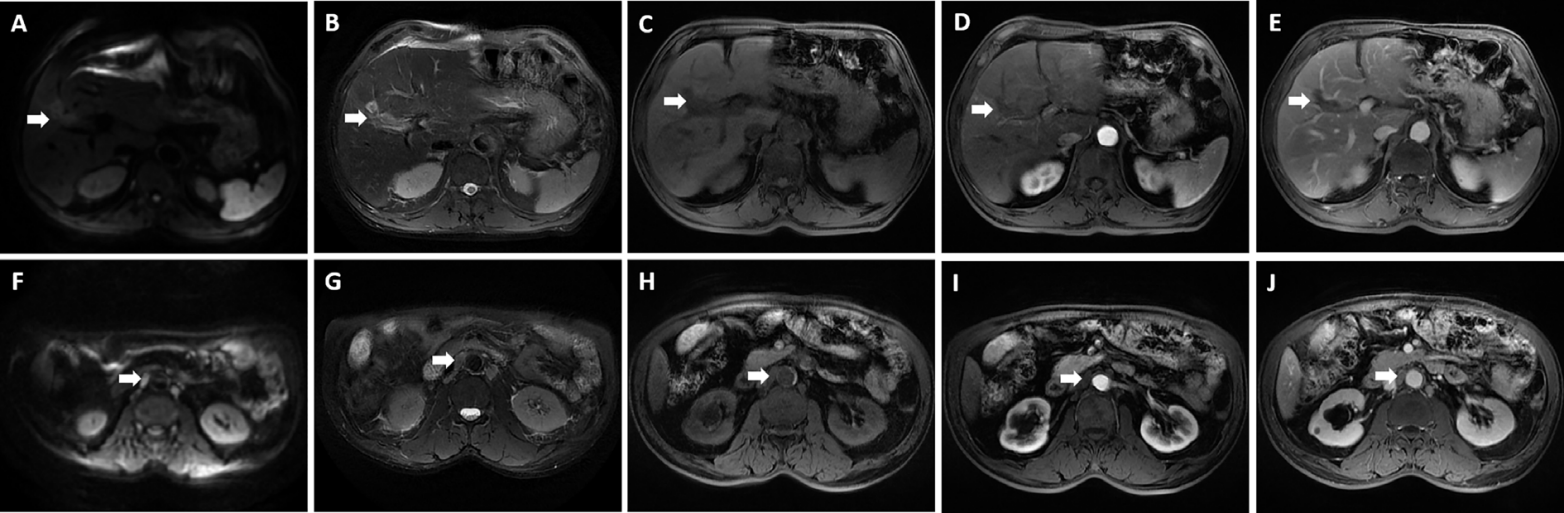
Preoperative magnetic resonance imaging was performed for the patient. The reduced lesion with clear boundary has low signal in T1-weighted imaging, high signal in T2-weighted imaging, and limited diffusion in diffusion-weighted imaging (DWI); enlarged hepatic portal and retroperitoneal lymph nodes were also revealed—the larger one was about 10 mm in diameter. (A, F) DWI; (B, G) T2-weighted imaging; (C, H) T1-weighted imaging; (D, I) The arterial phase; (E, J) The delayed phase. The white arrowheads direct the lesion or enlarged lymph nodes.
